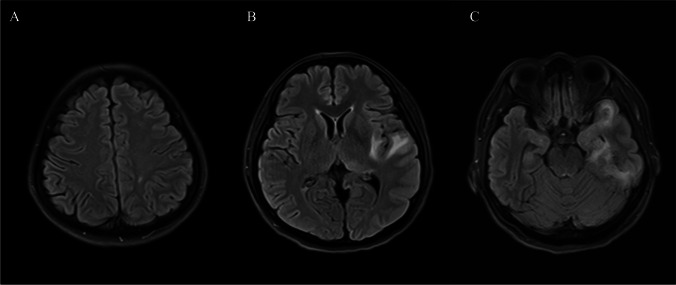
Typical imaging findings of COVID-19-related encephalitis. A–C: multiple abnormal signals were found in the frontal lobe, parietal lobe, temporal lobe, insula lobe, hippocampus, and thalamus on the head MRI T2 FLAIR phase
